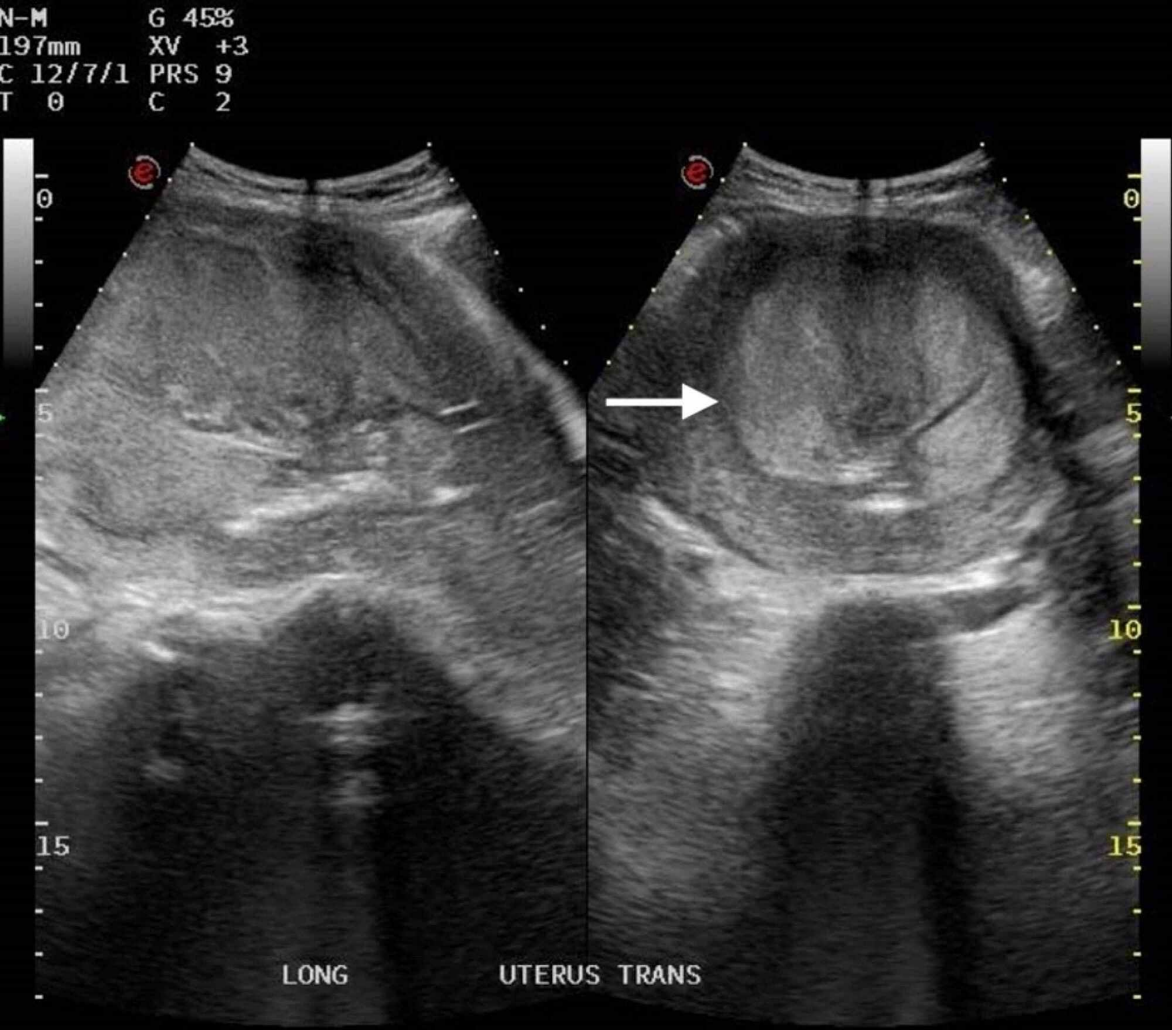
Ultrasound finding of an endometrial mass measuring 10 x 6.6 cm.
